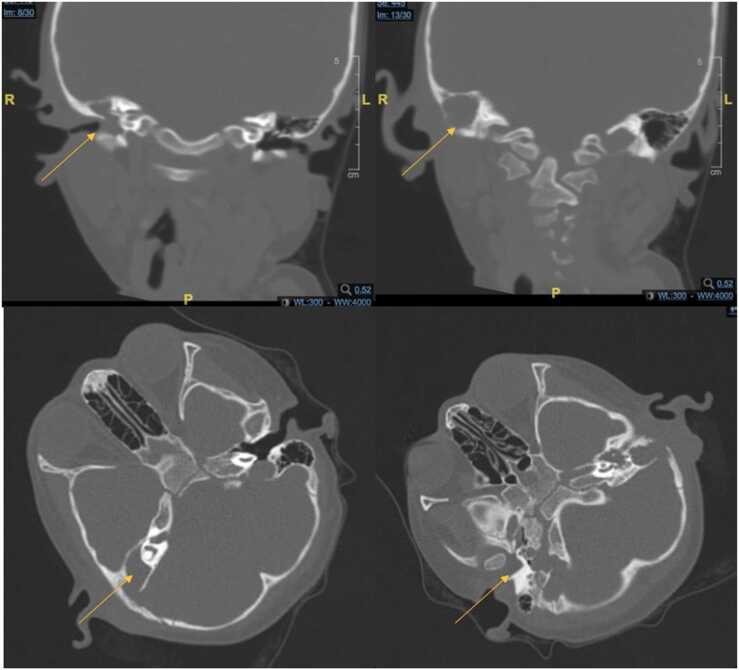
Head CT scan result revealed right osteomastoiditis with cholesteatome which destructed right mastoid antrum, right ear ossicle destruction, and also right posterior auricular abscess (yellow arrow).
